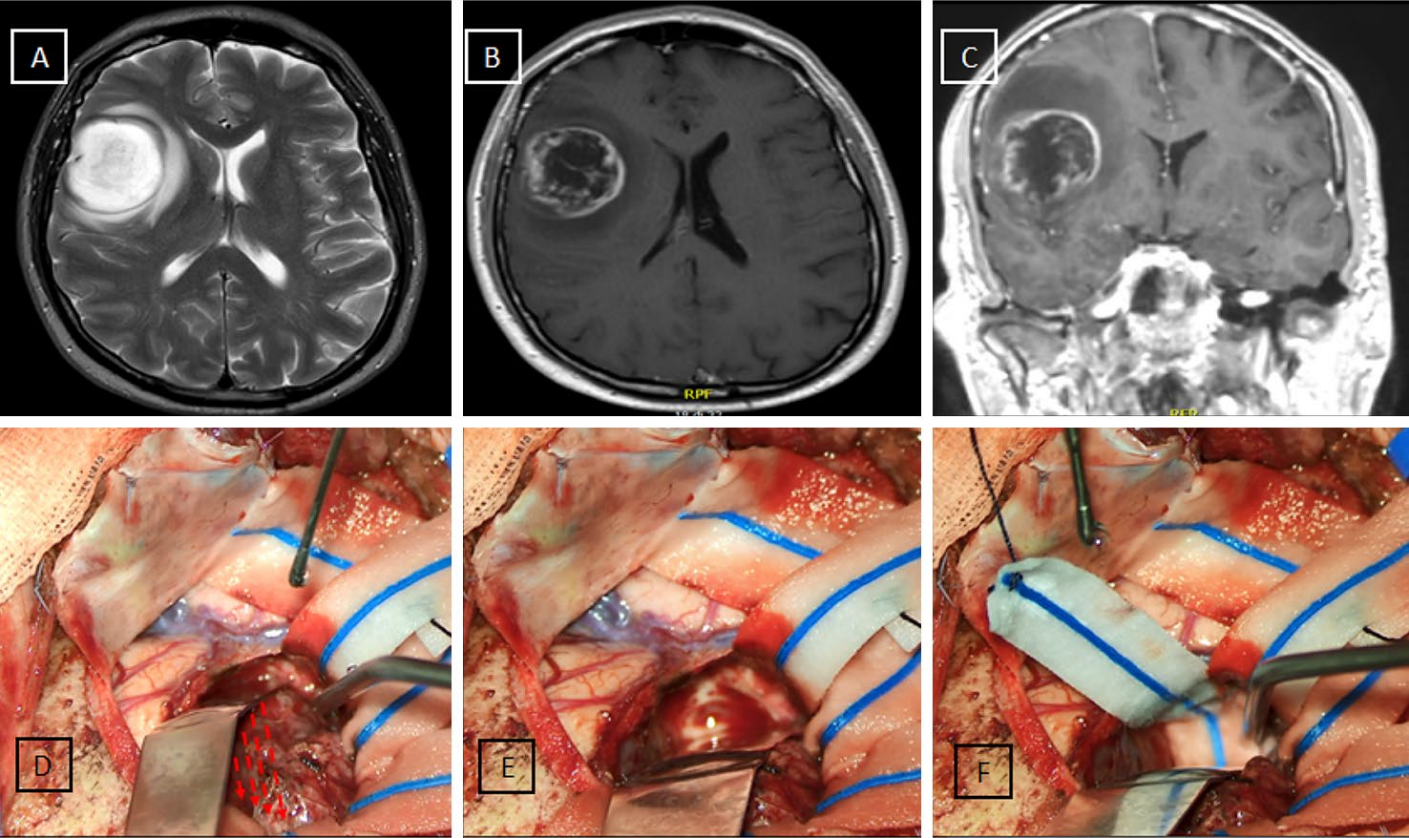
Shows the circumnavigation of a case of a right fronto-opercular GB: AT2-weighted preoperative MRI; B,C T1-weighted with gadolinium pre-operative brain MRI. D–F A dissection plane is found and delimited with cottonoid patties
